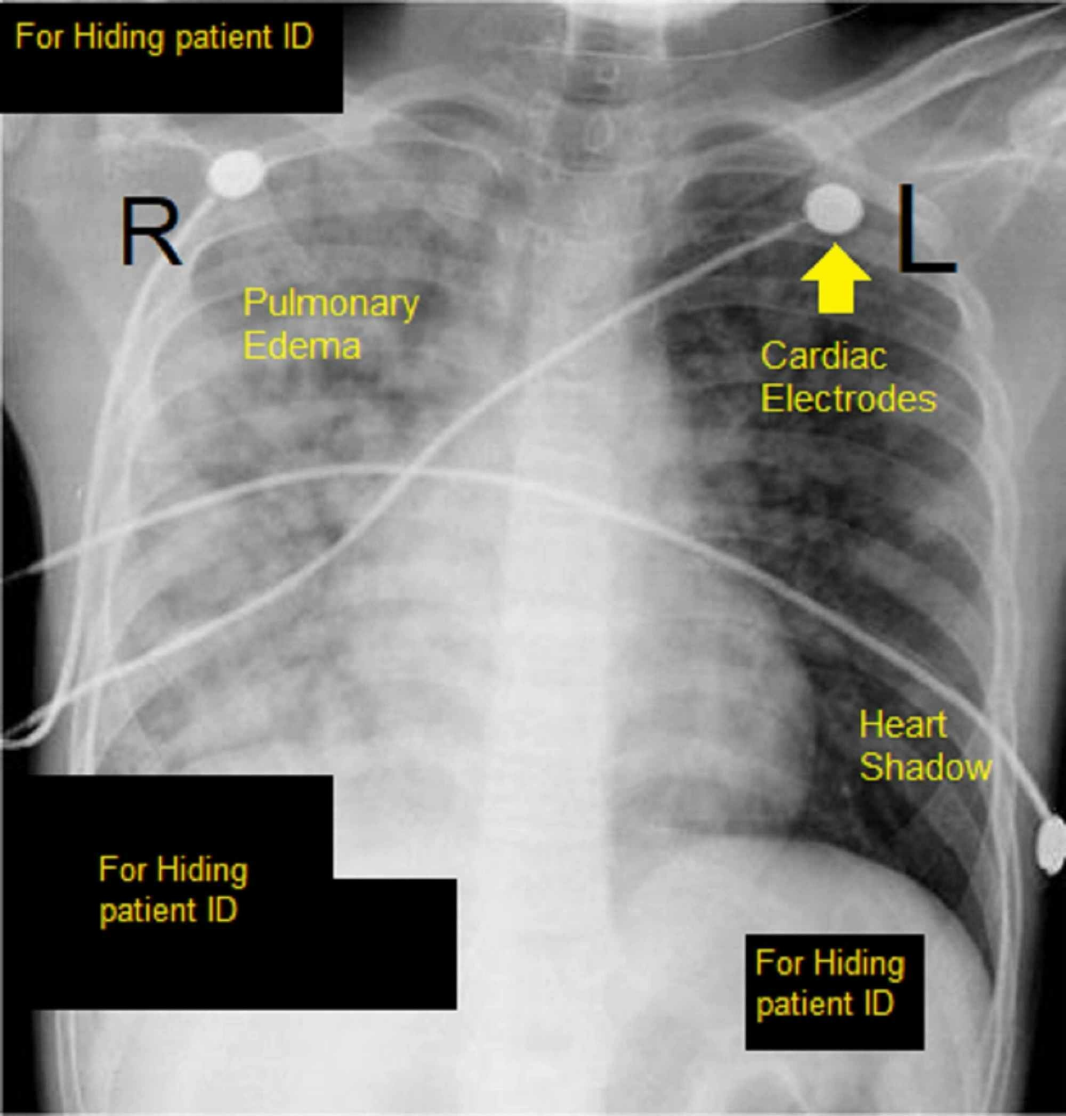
CXR showing pulmonary edema. CXR, chest X-ray
